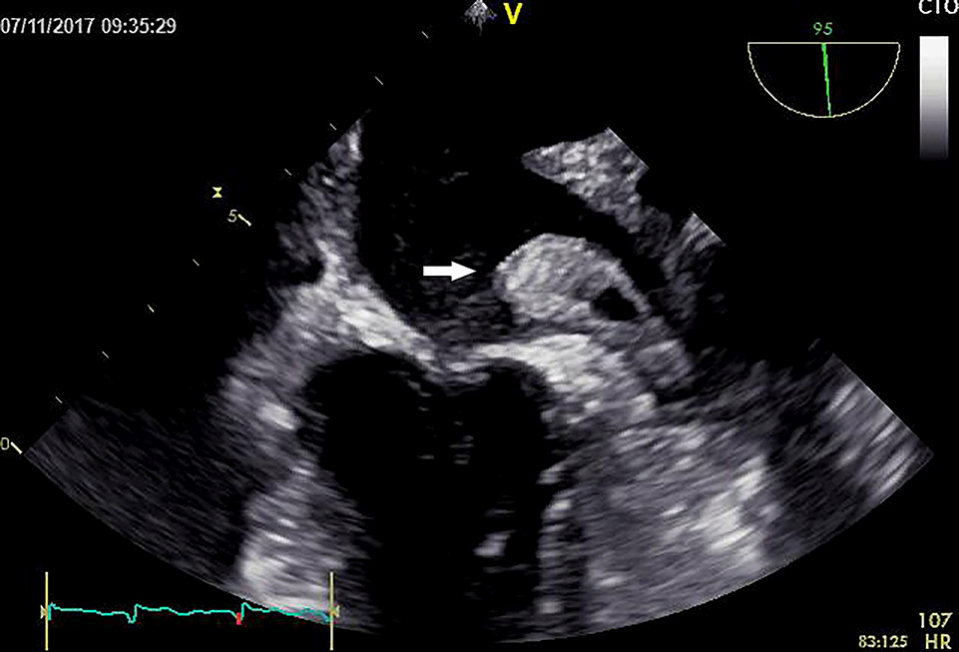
Transesophageal echocardiogram showed the large left atrial thrombus.
